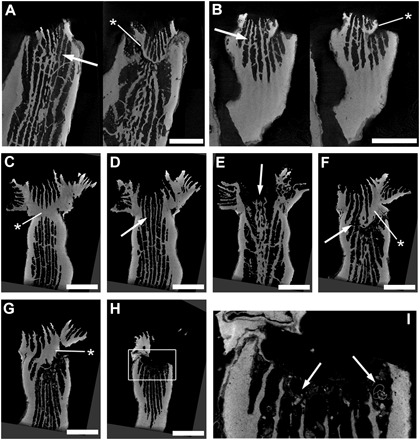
3D computed tomography sections in *C. caespitosa* calices showing rejuvenation. Septa and columnella partially connect through the abandoned and rejuvenated calices [arrows in (A) to (F)], while a new external wall is built [asterisks in (A) to (G)], leaving the rest of the skeletal structures and a portion of the original external wall outside the rejuvenated calix and characteristically marking the inner corallite structure. The new calix eventually recovers its original diameter, and the polyp starts budding (C to H). The partially abandoned calix, exposed to surrounding water, eventually fills with debris and foraminifera [arrows in (I), zoomed-in white rectangle of (H)]. Scale bars, 0.25 cm. Note that the sections belong to three corallites; (A) and (B) show two sections of two different corallites, while (C) to (I) belong to the same one.
